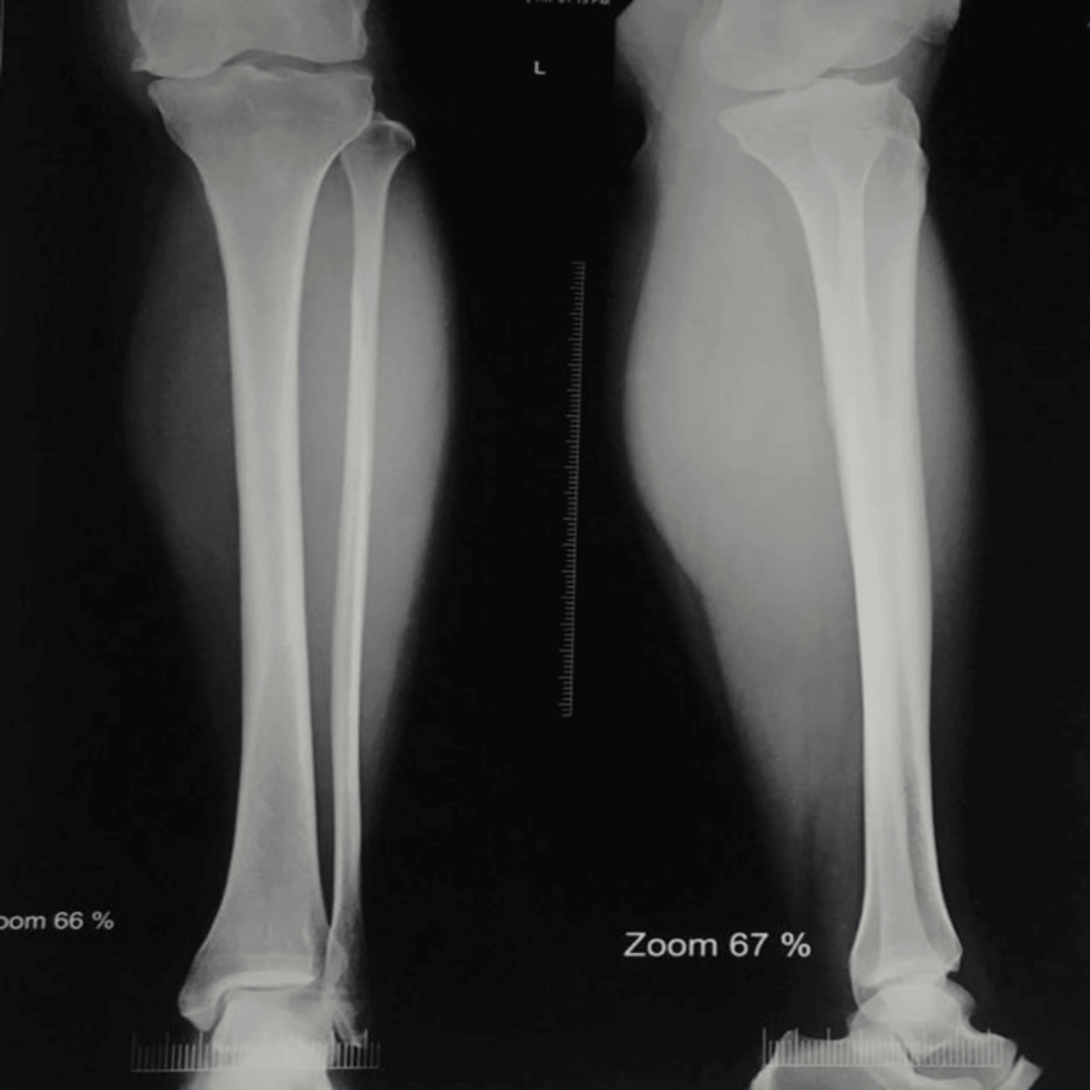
Preoperative full-length standing radiograph showing varus alignment and mechanical axis deviation toward the medial compartment
